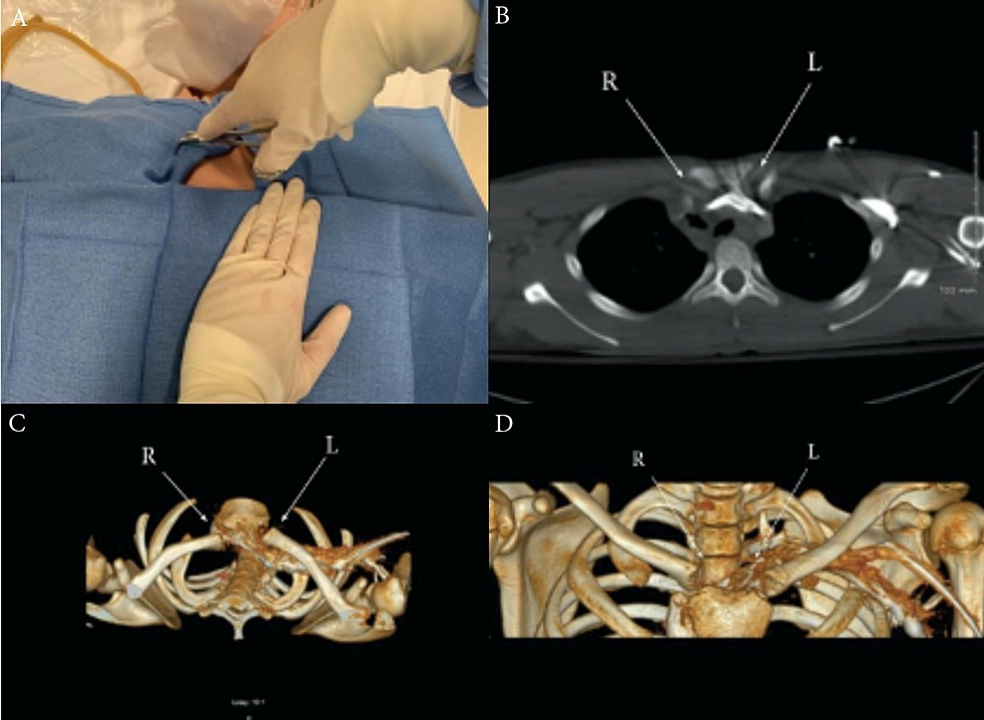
A) Reduction technique using percutaneous towel clamp forceps engaging the medial aspect of the dislocated clavicle while the other hand stabilizing the sternum. B) Axial CT showing anatomical reduction of posterior dislocation of the right sternoclavicular joint. C) Post-reduction axial CT reconstruction. D) Post-reduction coronal CT three-dimensional (3D) reconstruction. CT: computed tomography, R: right, L: left
